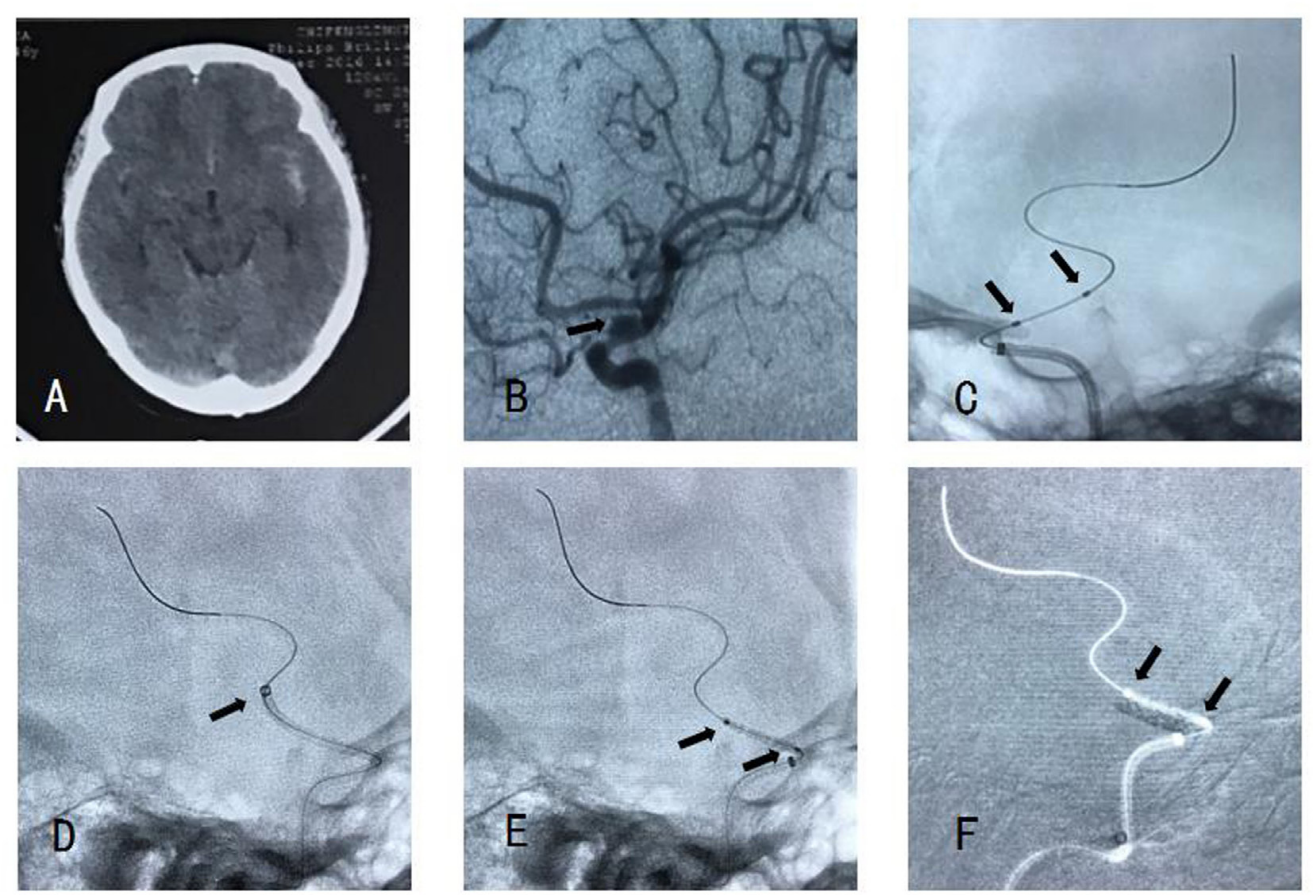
(A) Computed tomography showed subarachnoid hemorrhage. (B) Angiography showed a blood blister-like aneurysm. (C) There is no stent between two markers. (D) Navien accessed to distal of aneurysm neck enough. (E) Willis covered stent (WCS) accessed to aneurysm neck smoothly. (F) The WCS was expanded successfully and covered the aneurysm neck.
